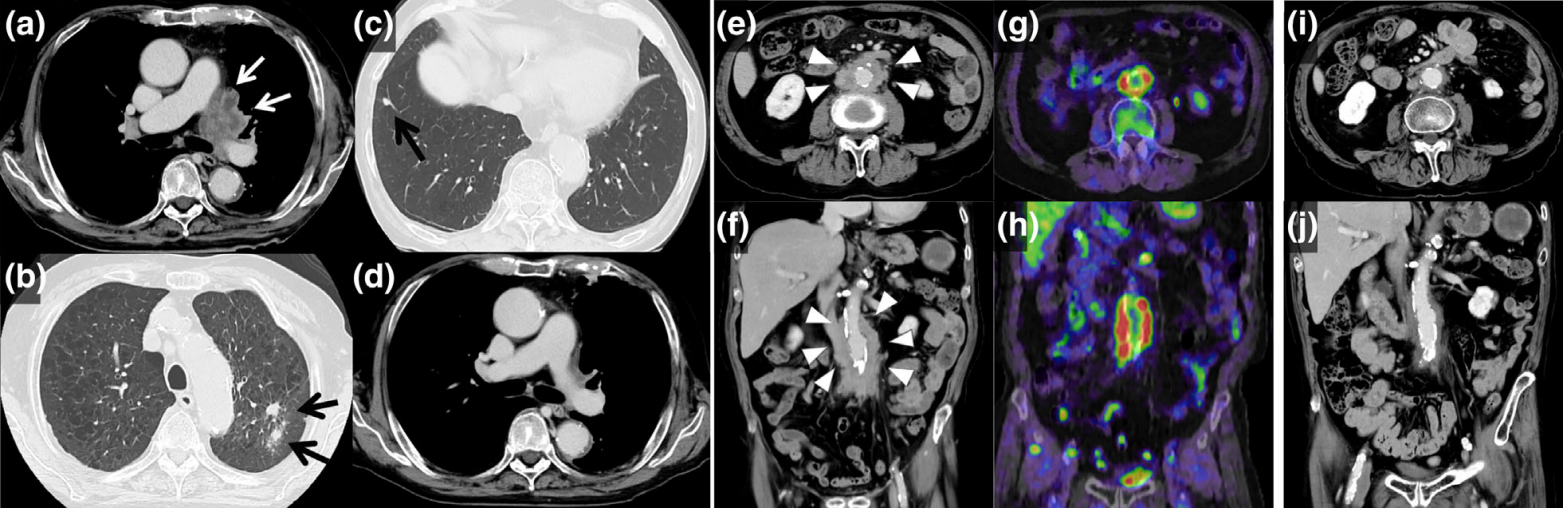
Imaging studies of the chest with the relevant timeline. (a–c) Chest contrast‐enhanced computed tomography (CT) scan revealed a tumor lesion that was fused between the left hilum and mediastinum (white arrow). The tumor enveloped the left pulmonary artery, and nodules were detected in both lung fields (black arrow). (d) A chest contrast‐enhanced CT scan taken eight months after the initiation of treatment showed a reduction in the tumor size around the left pulmonary artery. (e: cross‐section, f: coronal‐section) A pelvic contrast‐enhanced CT scan taken at the same time as that of (d) revealed soft tissue shadows around the abdominal aorta that had increased in size (arrowheads). Soft tissue shadows around the bladder and seminal vesicles had also increased in size. (g: cross‐section, h: coronal‐section) A positron‐emission tomography (PET‐CT) scan taken at the same time as that of (d) showed accumulation around the abdominal aorta and bladder. (i: cross‐section, j: coronal‐section) One month after the initiation of steroid treatment, a CT scan showed a reduction in the soft tissue shadow around the abdominal aorta.
